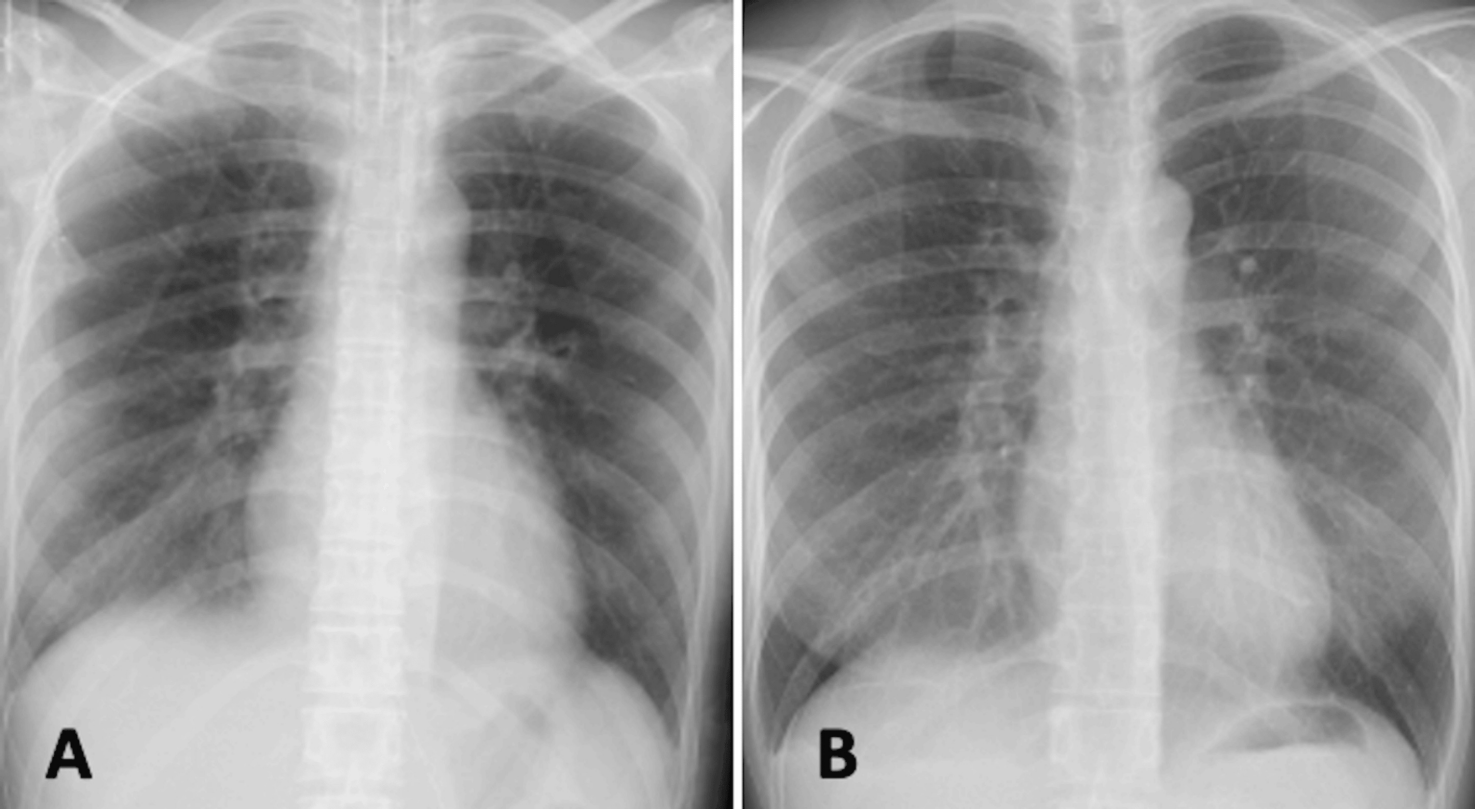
Postoperative chest radiographs (A) Postoperative day (POD) 1: Pulmonary edema markedly improved following mechanical ventilation and diuretic therapy. (B) POD 4: Pulmonary findings nearly normalized.
